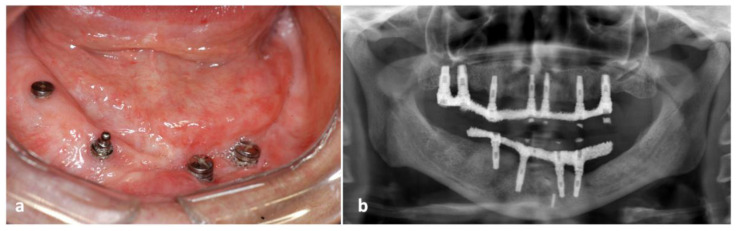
Clinical condition with 10 years of follow-up (a) and radiographic aspects after 15 years of follow-up (b). Clinical and radiographic features showing no evidence of recurrence or disease relapse.
